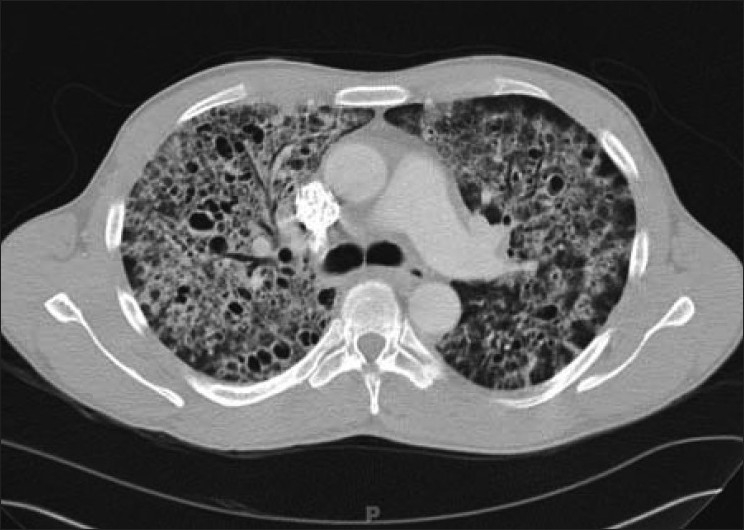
Pneumocystis carinii* pneumonia (PCP). High-resolution computed tomography showing the hallmark of PCP in a clinical setting of immune compromise. Note the ground-glass attenuation with a geographic or mosaic distribution
